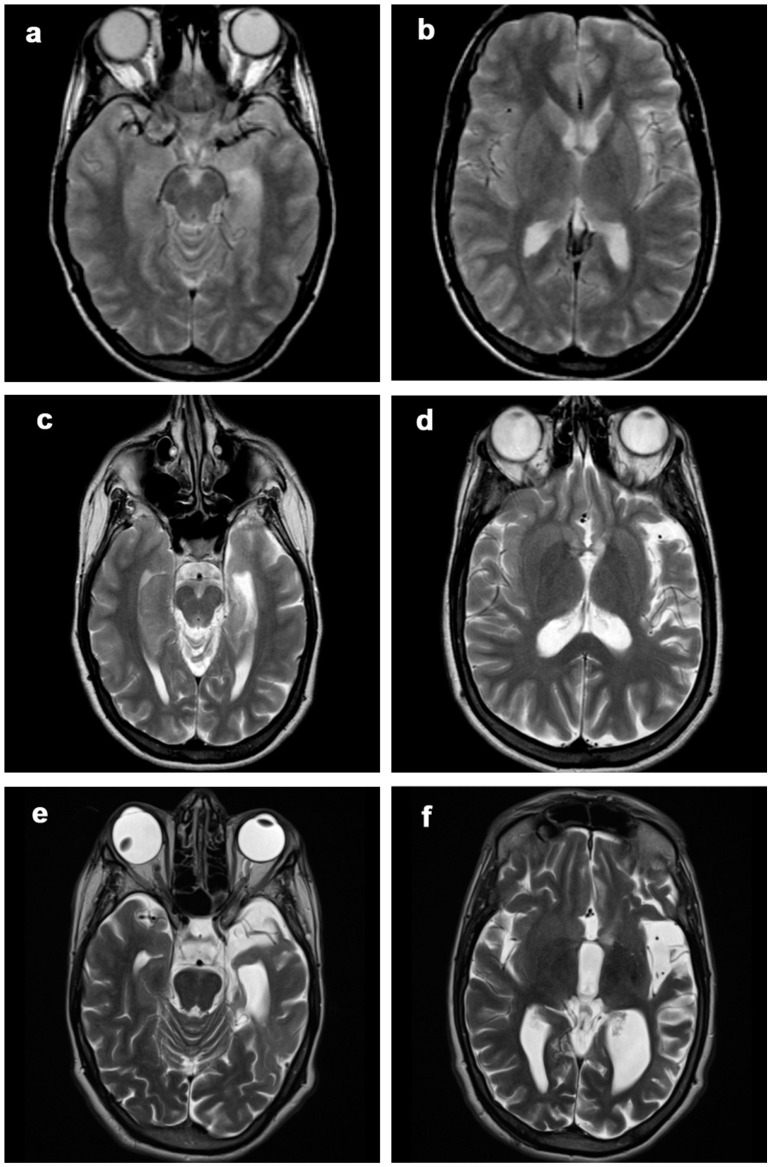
Patient 1, progression of the disease in 21 years. Axial (a–f) T2-weighted images at (a,b) 2 years, (c,d) 12 years and (d,e) 21 years from clinical onset. The disease slowly progressed over time, involving mainly frontobasal, insular, perisylvian and temporal left structures. At first MRI scan, only subtle atrophic changes could be seen in the perisylvian region (b).
